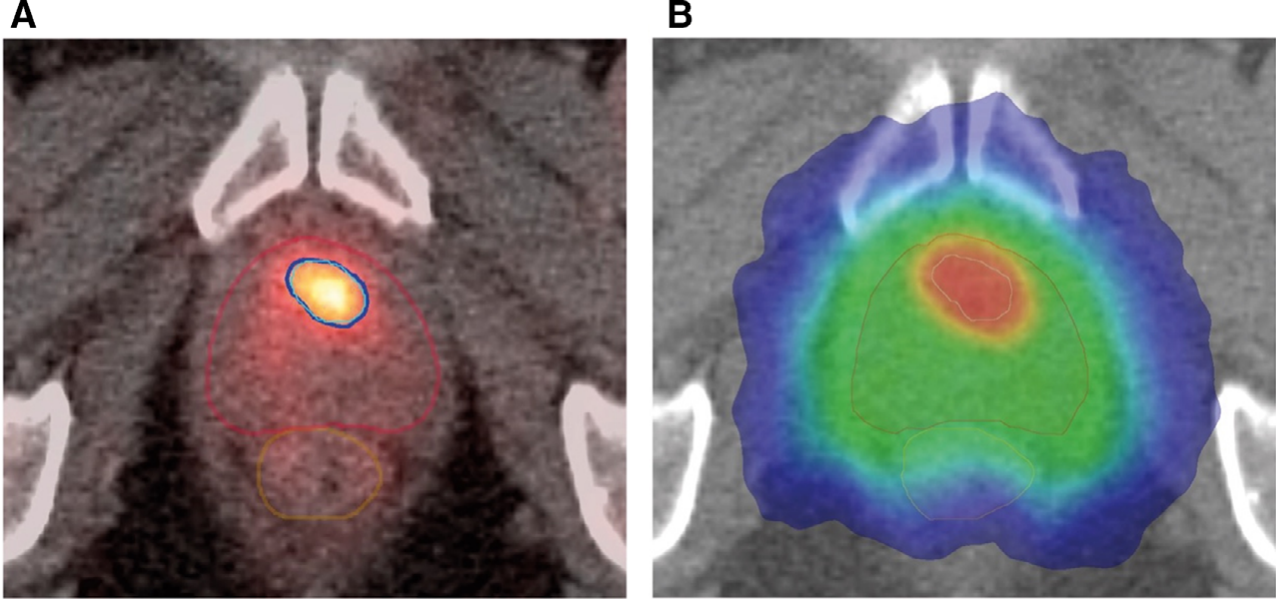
PSMA‐PET‐based focal boosting in prostate cancer. (A) Axial PSMA‐PET‐CT slice showing the contours of the prostate (red), GTV (cyan), rectum (brown), and the 50 Gy isodose (5 fractions of 10 Gy; marine blue). (B) Corresponding CT slice with color wash isodose curve showing conformal dose shaping to the prostate (clinical tumor volume) treated to 35 Gy in five fractions of 7 Gy and intraprostatic tumor (GTV) with sparing of the rectum and urethra. The intraprostatic lesion (cT1c, Gleason 3 + 4 = 7, iPSA = 16.6 ng·mL^−1^) is located in the left transition zone. The patient participated into the multicenter prospective phase II hypo‐FLAME study (NCT02853110, ClinicalTrials.gov).
